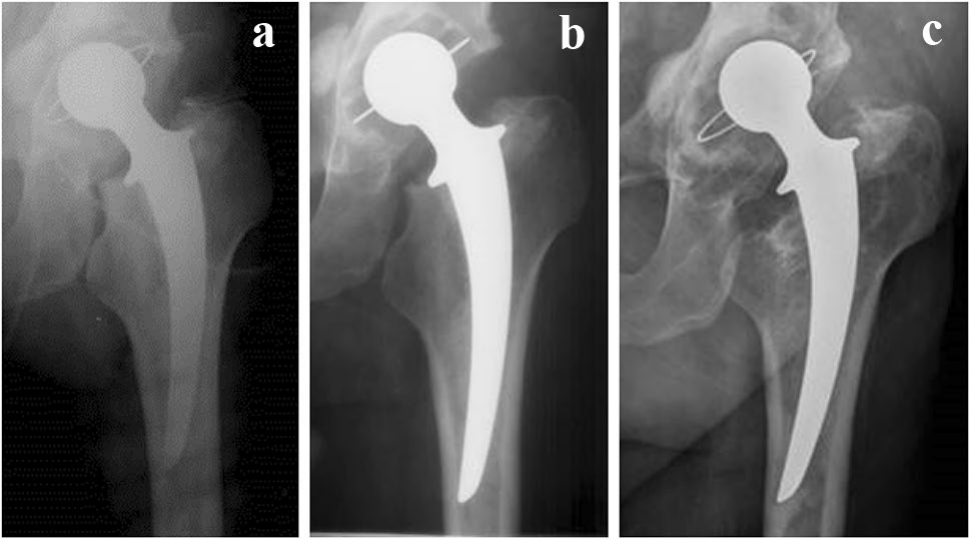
X-ray image of Alivium Charnley–Muller prosthesis after the primary operation (a), 3 months post-op (b), and 31 years post-op, preceding the revision (c)
